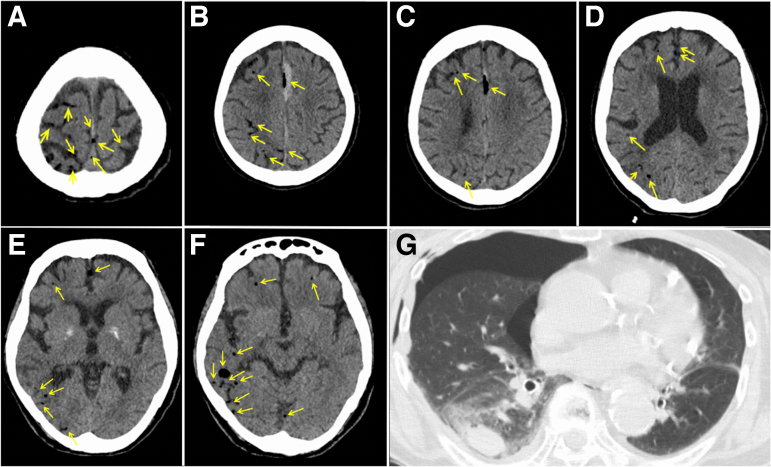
Computed tomographic images at the time of visit. (A–F) The air was in the right side of the brain and on the brain surface. Yellow arrows indicate air. (G) Right traumatic hemopneumothorax, pulmonary contusion, and multiple rib fractures were observed.
